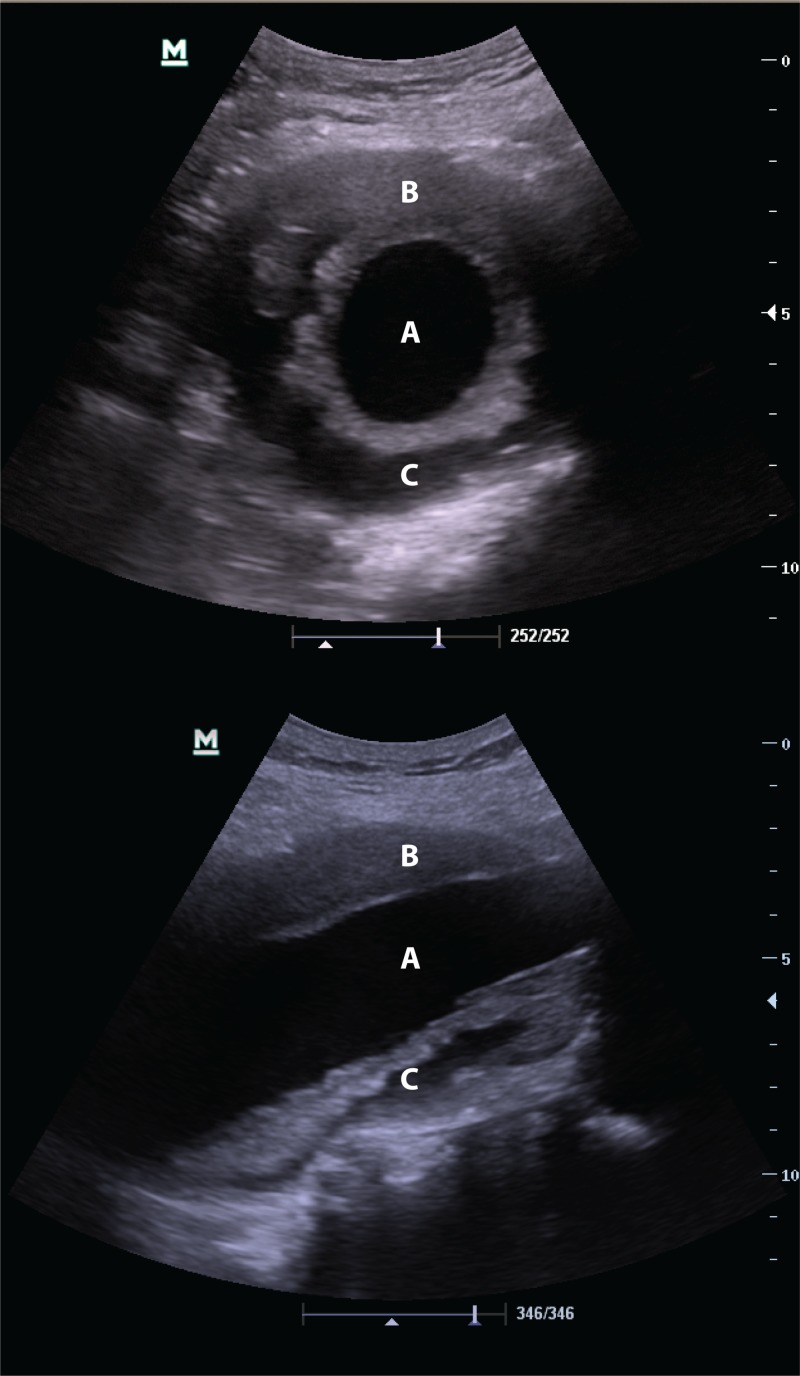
Point-of-care ultrasound images of the abdominal aorta Top: transverse section; Bottom: longitudinal section Both the transverse and longitudinal views of the abdominal aorta show a large abdominal aortic aneurysm with a central lumen (A), an area of stable thrombus (B), and areas of dissection or hemorrhage within the thrombus in the lateral and posterior aspects of the aorta (C).
